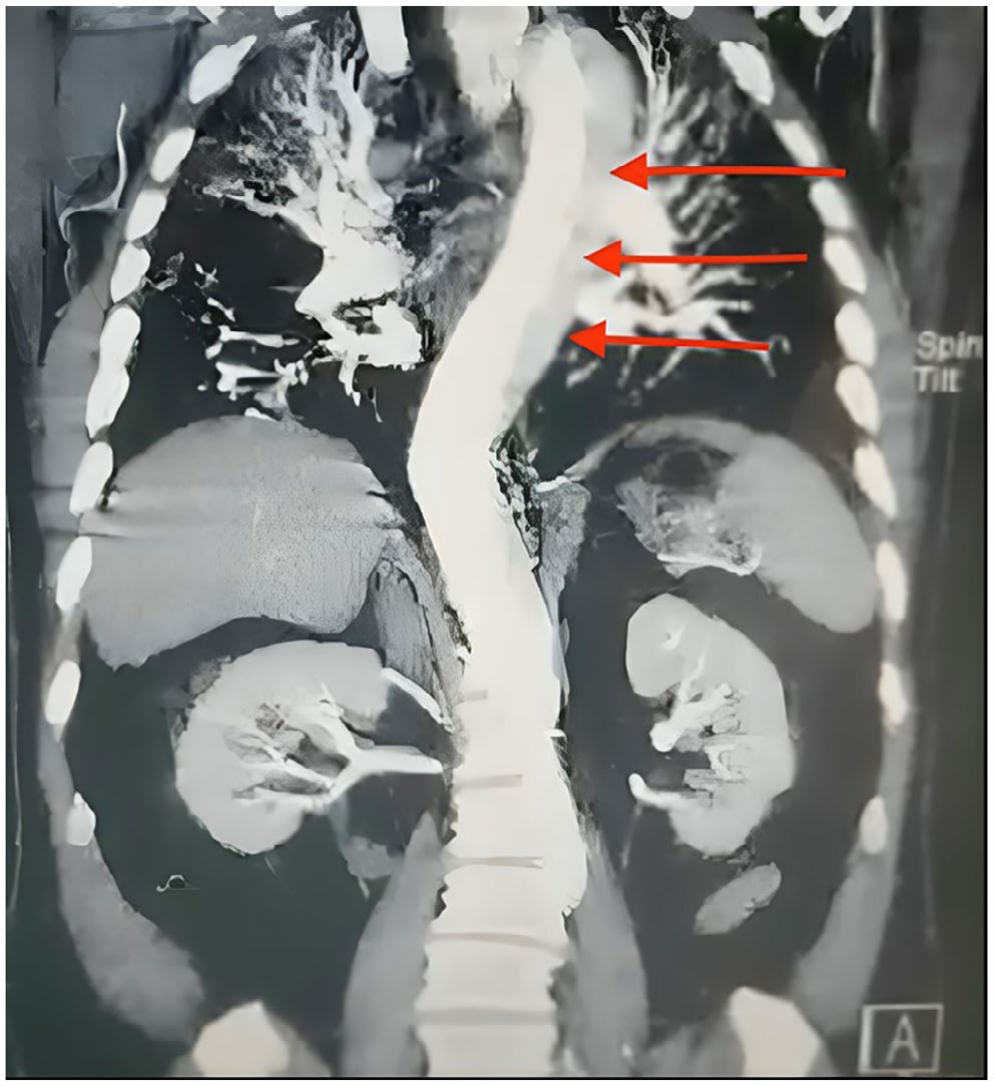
2D CT angiography of the chest and abdomen showing aortic dissection (red arrows). The dissection involves Stanford type A, where the intimal layer of the aorta separates, allowing blood to flow between the layers of the vessel wall. This dissection extends from the aortic root through the ascending aorta and into the abdominal aorta.
